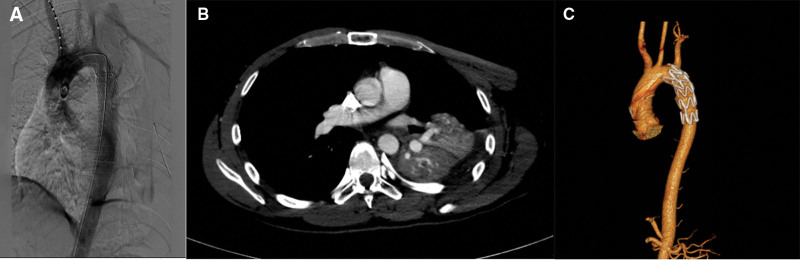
A 35-year-old male with BTAI caused by a car accident. (A) The tear was located at the lesser curvature of descending aorta seen by preoperative CTA. (B) The postoperative reexamination showed that the lesion was well sealed without endoleak, and the LSA had no obstruction. (C) The stent was in good shape and position with no endoleak and no obstruction of the LSA during the follow-up 5 years after the operation. BTAI = blunt traumatic aortic injury, CTA = computed tomography arteriography, LSA = left subclavian artery.
